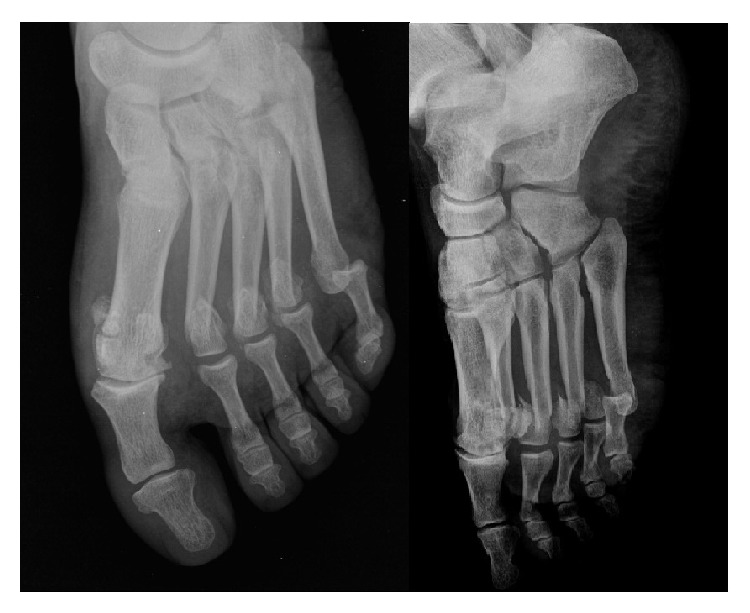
The X-ray of the patient on first examination.
